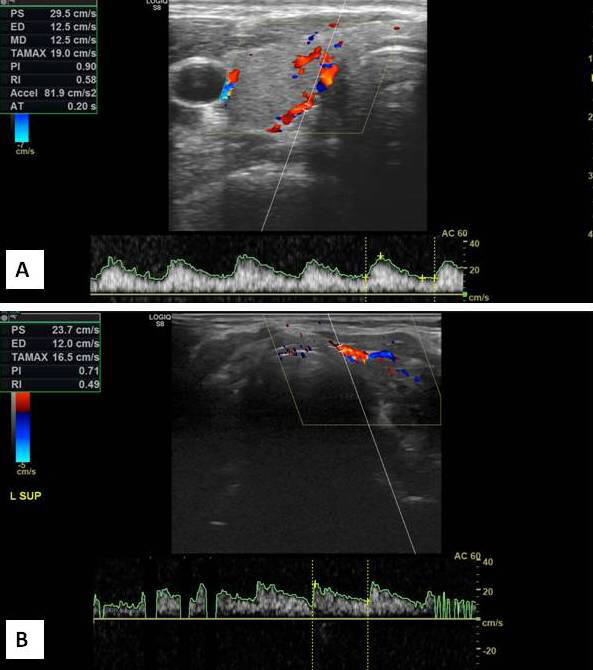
A. 55-year-old, male, normal thyroid tissue. Doppler examination of the right superior thyroidal artery. B. 56-yearold, female, Hashimoto’s thyroiditis. Doppler examination of the left superior thyroidal artery. Compared with normal tissue, PI and RI values and Vmax and Vmin decreased in accordance with the increased tissue resistance (Doppler indices are on the images).
